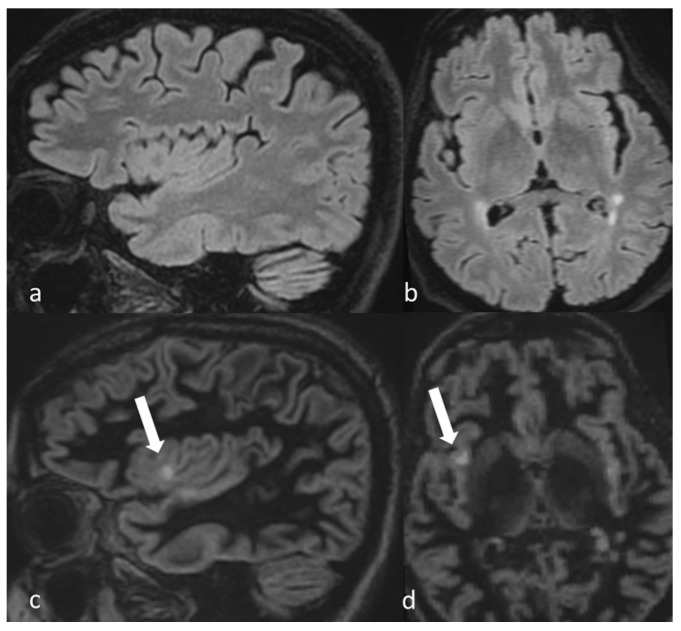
(a,b) The right insula cortical lesion (arrow) is unapparent on sagittal and axial FLAIR; (c,d) the same cortical lesion is well detected on sagittal and axial DIR images.
